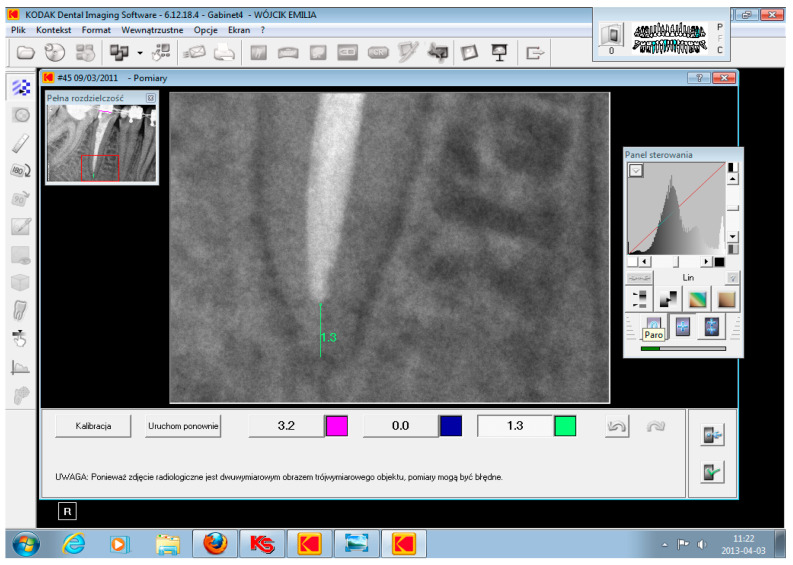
The screenshot of intraoral periapical radiograph (root’s apex magnification) of endodontically treated tooth presenting the measurement of the root canal filling range.
